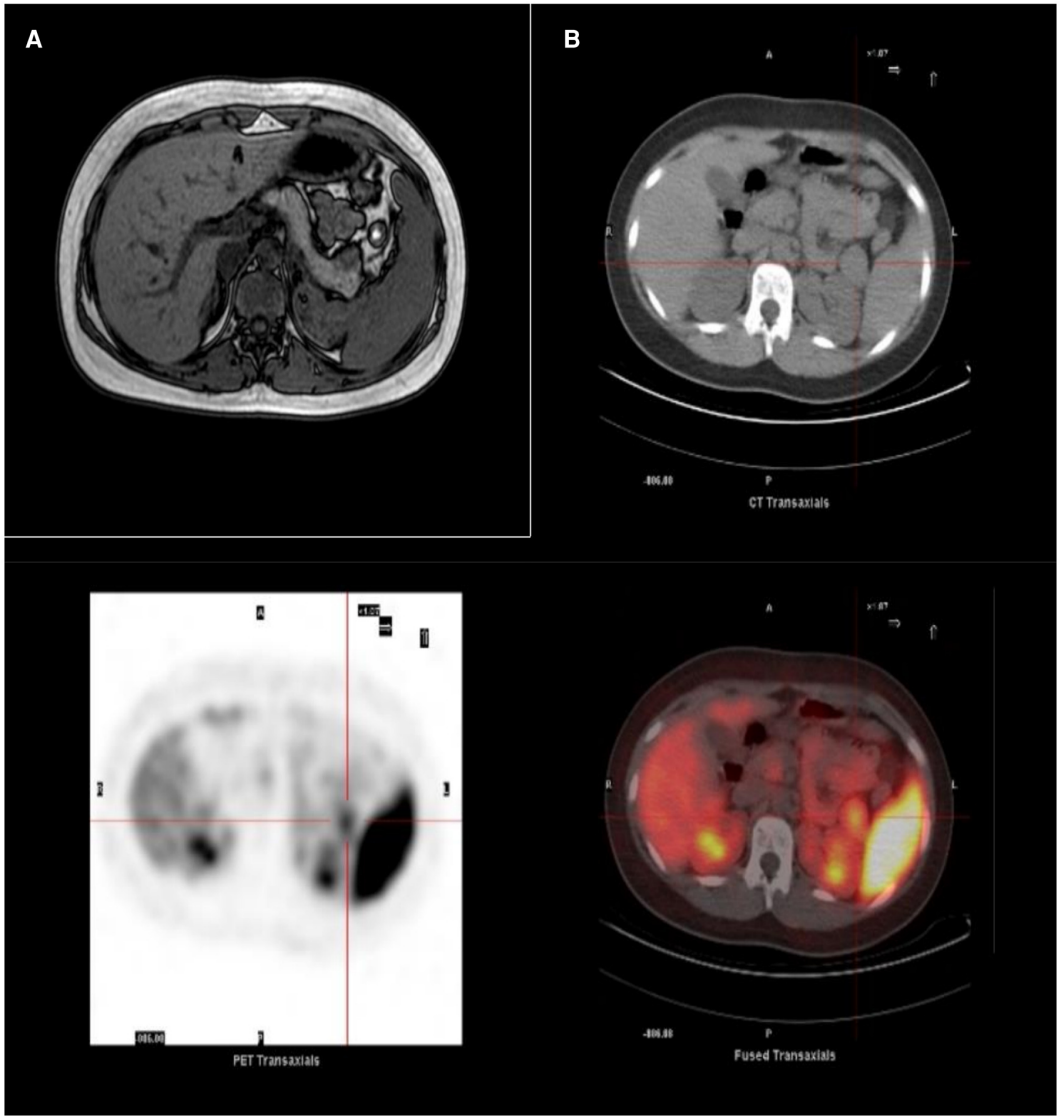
Imaging of insulinoma. (A) MRI showing a lesion in the pancreatic tail. (B) ^68^Ga-DOTATOC PET/CT scan showing selective radiotracer uptake by the lesion consistent with insulinoma.
